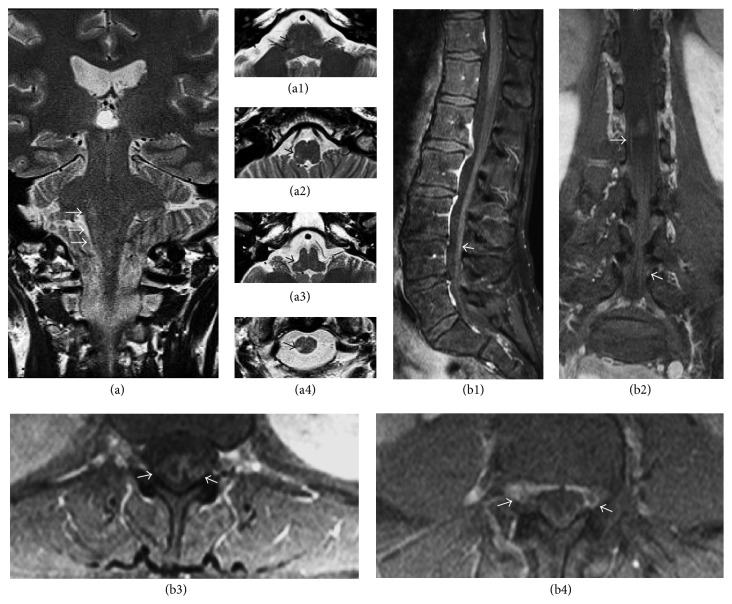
(a) Coronal and axial MRI sections of the brainstem to spinal cord showed small hypersignal-T2 in the right dorsolateral mid-pons (a1) and from the right dorsolateral aspect of the pontomedullary region (a2) to the right dorsolateral aspect of the upper cervical cord (a3-a4), along the course of the principle sensory nucleus and spinal nucleus of the right trigeminal nerve. Sagittal (b1), coronal (b2), and axial (b3-b4) spinal MRI showed enhancement of the nerve roots along the spinal cord.
